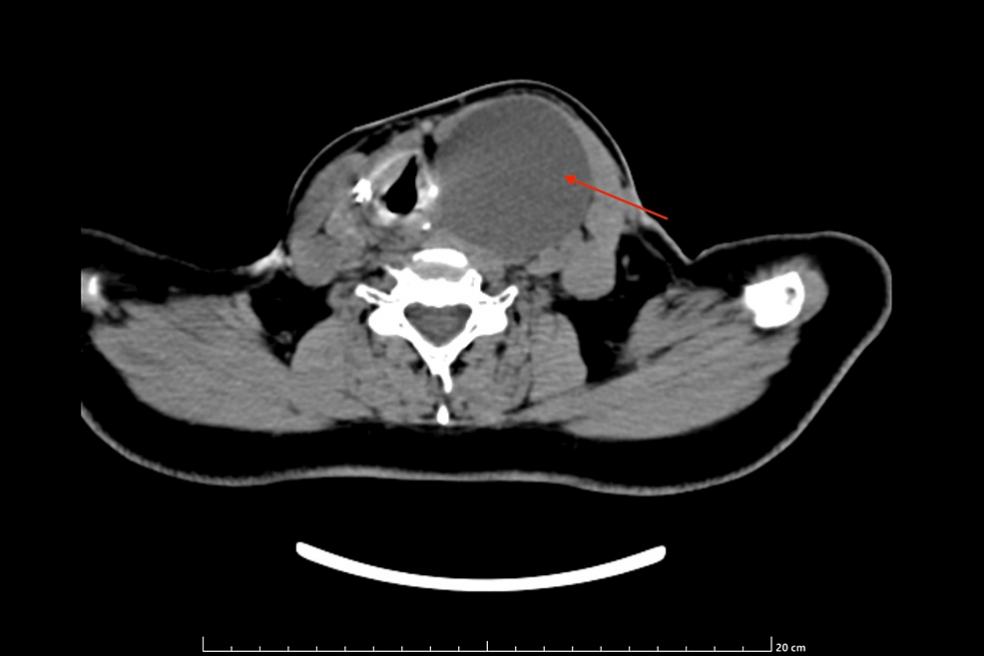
CECT of the neck showing a large, well-defined, lobulated, heterogeneously-enhancing solid cystic lesion in the left lobe of thyroid CECT: Contrast-enhanced computed tomography
